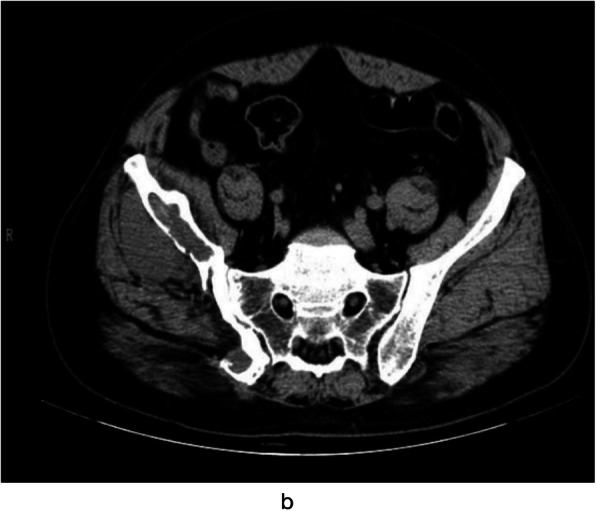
CT plain scan, showing cystic expansive bone destruction of the right iliac bone, right iliac bone and surrounding soft tissue, right pelvic wall and right gluteal muscles
